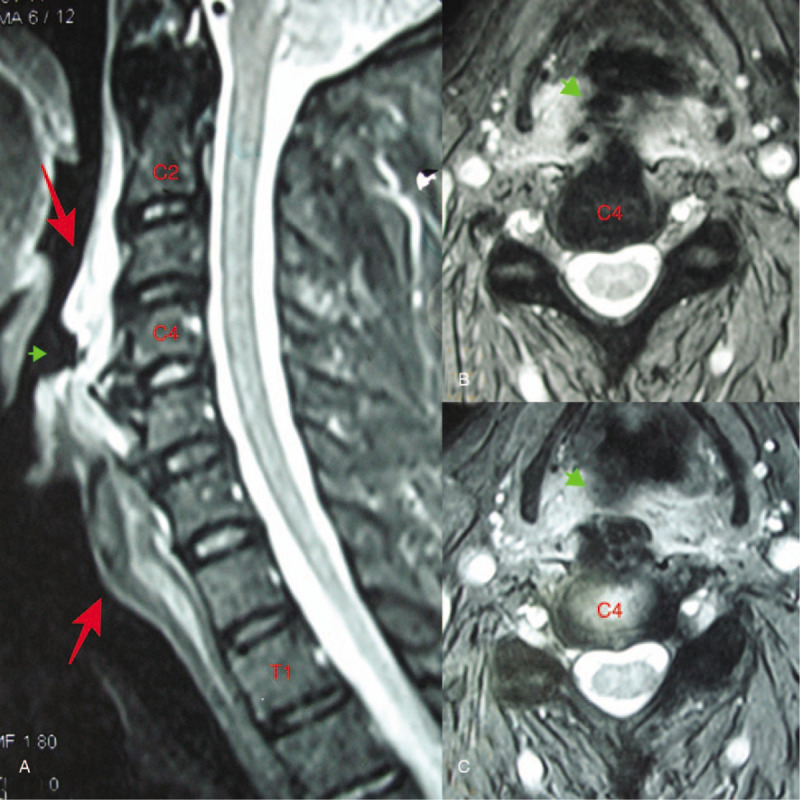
(A) Sagittal cervical spine MRI showed that an increased diffusion signal in the anterior soft tissue from the C2 to T1 cervical vertebral bodies on T2-weighted images and a small niche (arrowhead) on the posterior wall of the pharynx. (B and C) Axial MRI slices also showed a small niche (arrowhead) on the posterior wall of the pharynx. MRI = magnetic resonance imaging.
